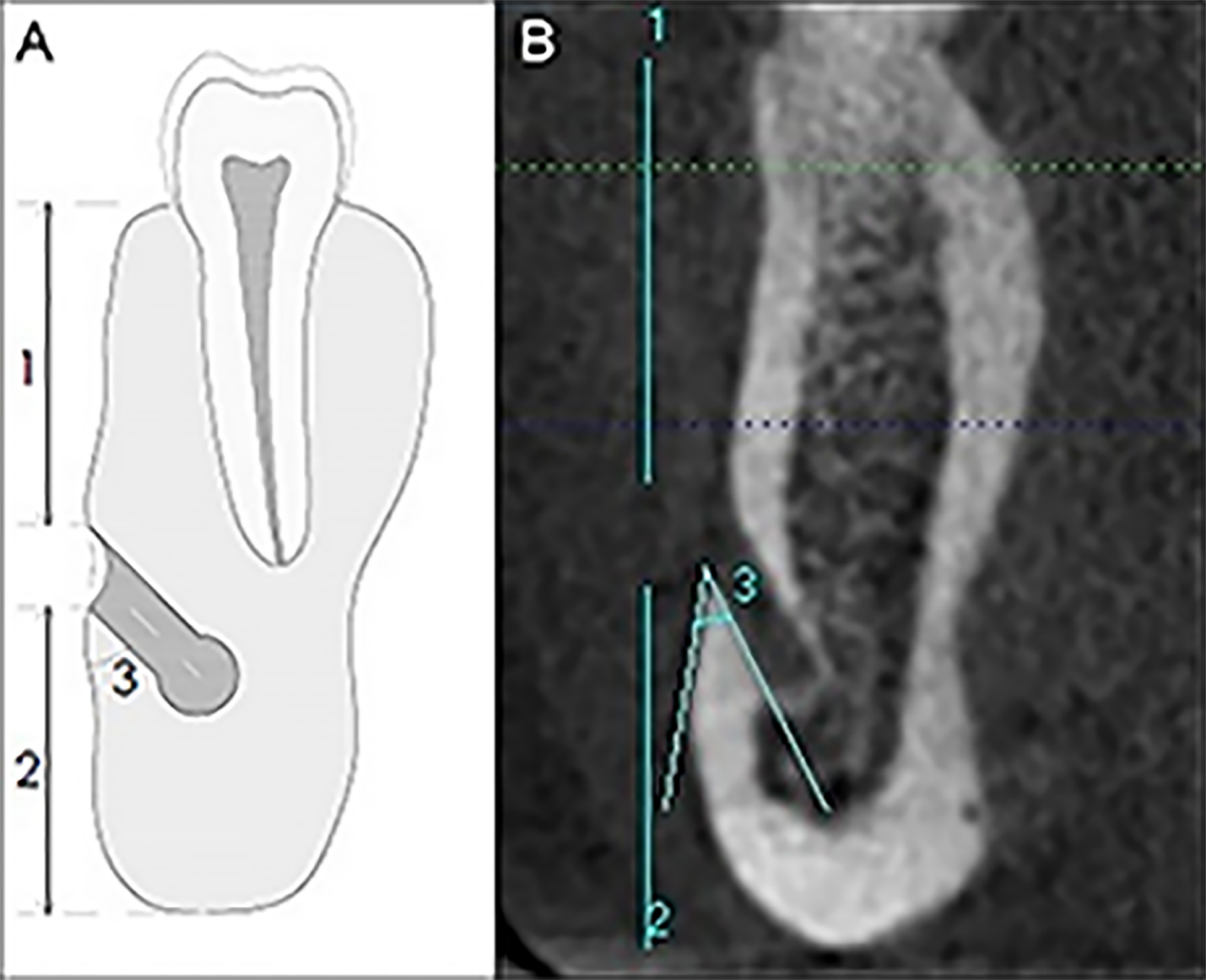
Location of the MF. (A) MF-MSB distance (1), MF-MIB distance (2), Emerging angle of the MF (3). (B) CBCT cross-sectional reconstructed slices of the mandible to show MF-MSB and MF-MIB distances, and emerging angle.
